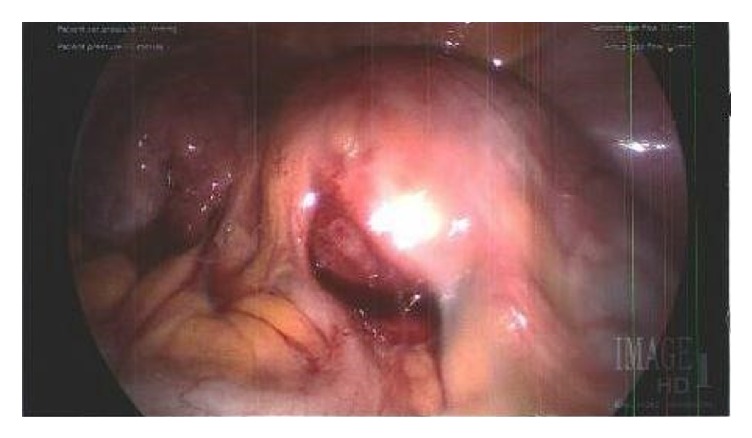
Ultrasound of heterogenous right adnexal mass measuring 12.6 × 8.2 × 7.9 cm, described as part solid-part cystic in nature.
